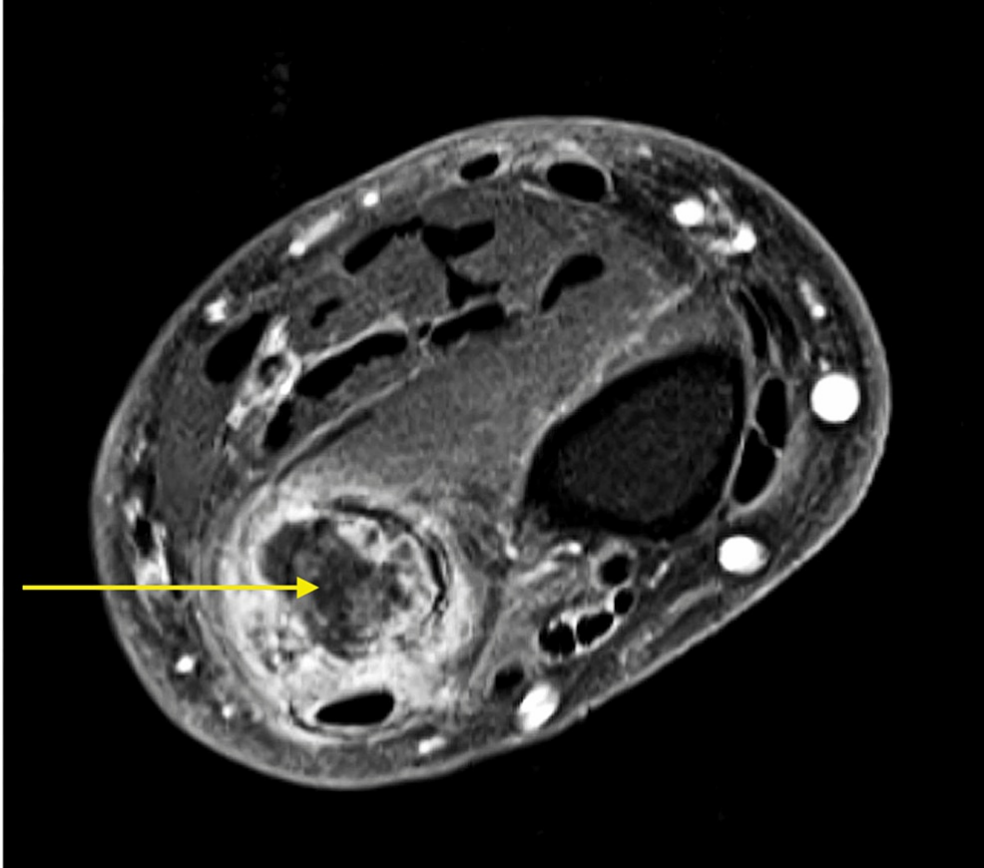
Pre-operative T1 weighted MRI of the right forearm with fat saturation post-contrast in axial view. Shows lobular enhancement, which is diagnostic for a cartilage tumor.
